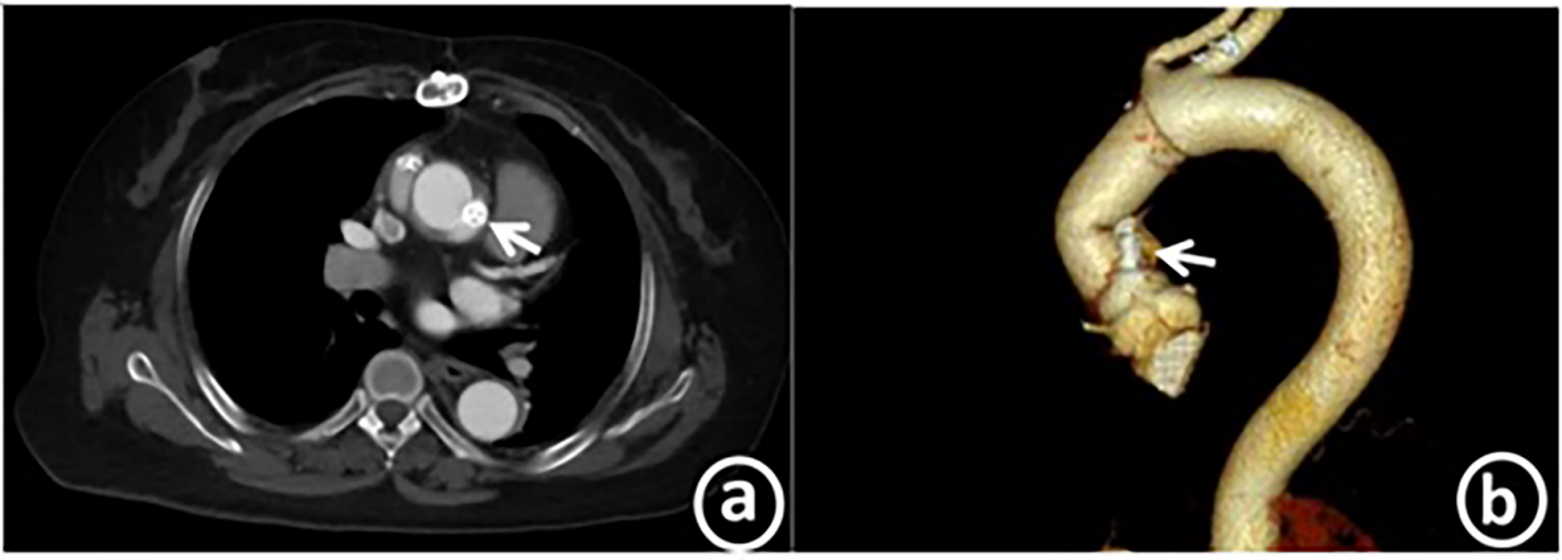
Post-operation, coronary CTA reveals the echo of the occluder, which is in a good position and has excellent molding. Panel a shows the axial plane, while panel b displays the curved planar reformation.
